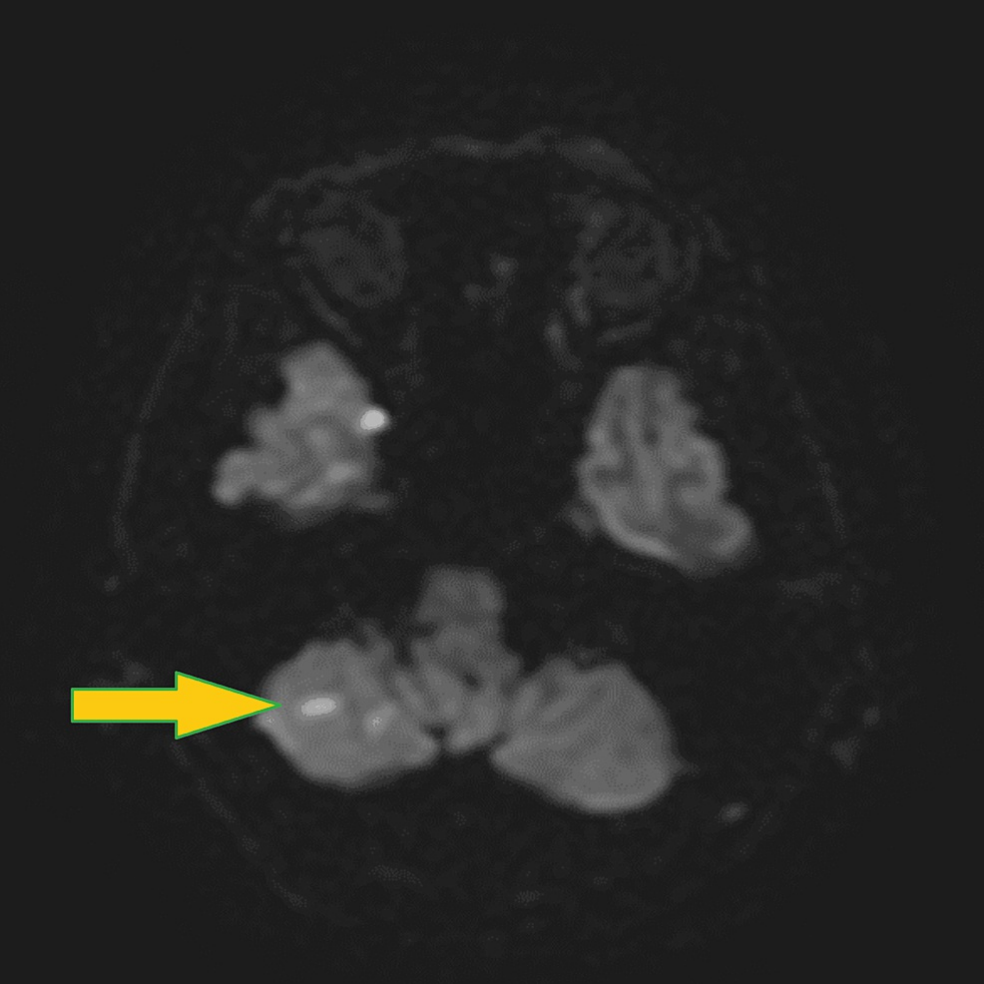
Brain MRI without contrast DWI sequence shows the cerebellar infarcts (arrow). MRI: magnetic resonance imaging, DWI: diffusion-weighted imaging
